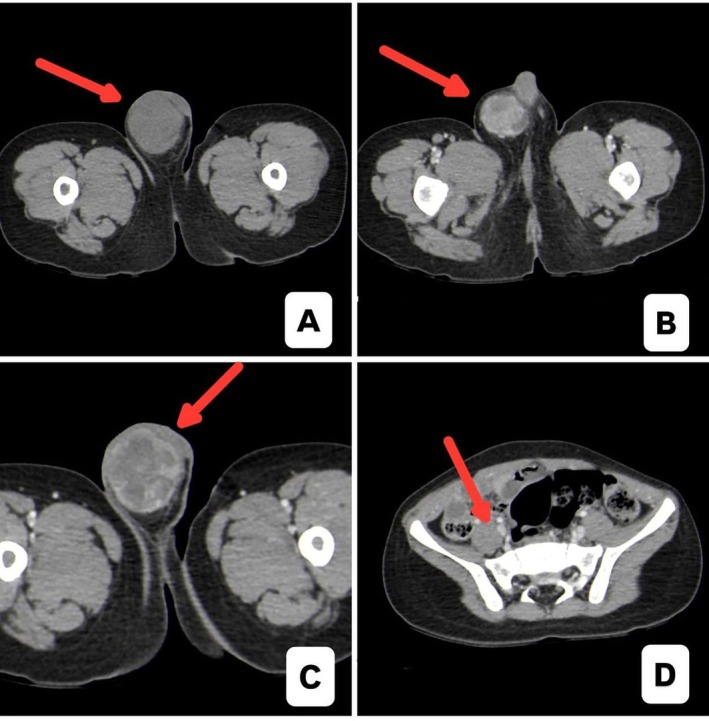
(A–C) Axial CT images of the pelvis/scrotum (A: Noncontrast, B, C: Contrast‐enhanced) demonstrate a well‐defined mass measuring approximately 6 × 3.4 × 3.2 cm occupying the right scrotum and extending along the right spermatic cord. (D) Contrast‐enhanced image highlights prominent right inguinal and right external iliac lymph nodes, measuring up to 0.6 cm, as well as mildly prominent left external iliac (0.6 cm) and right common iliac (0.5 cm) nodes.
